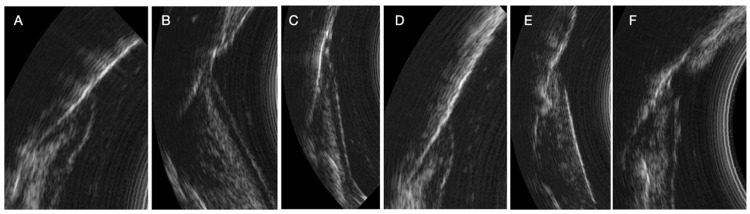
Variations in periodontal ultrasound images. (A) Reference image with normal structures. (B) Presence of deep inflammation with visible connective tissue junction. (C) Presence of deep inflammation without visible connective tissue junction. (D) Presence of supragingival calculus. (E) Presence of subgingival calculus. (F) Presence of calculus above and below the gingival surface and deep inflammation.
